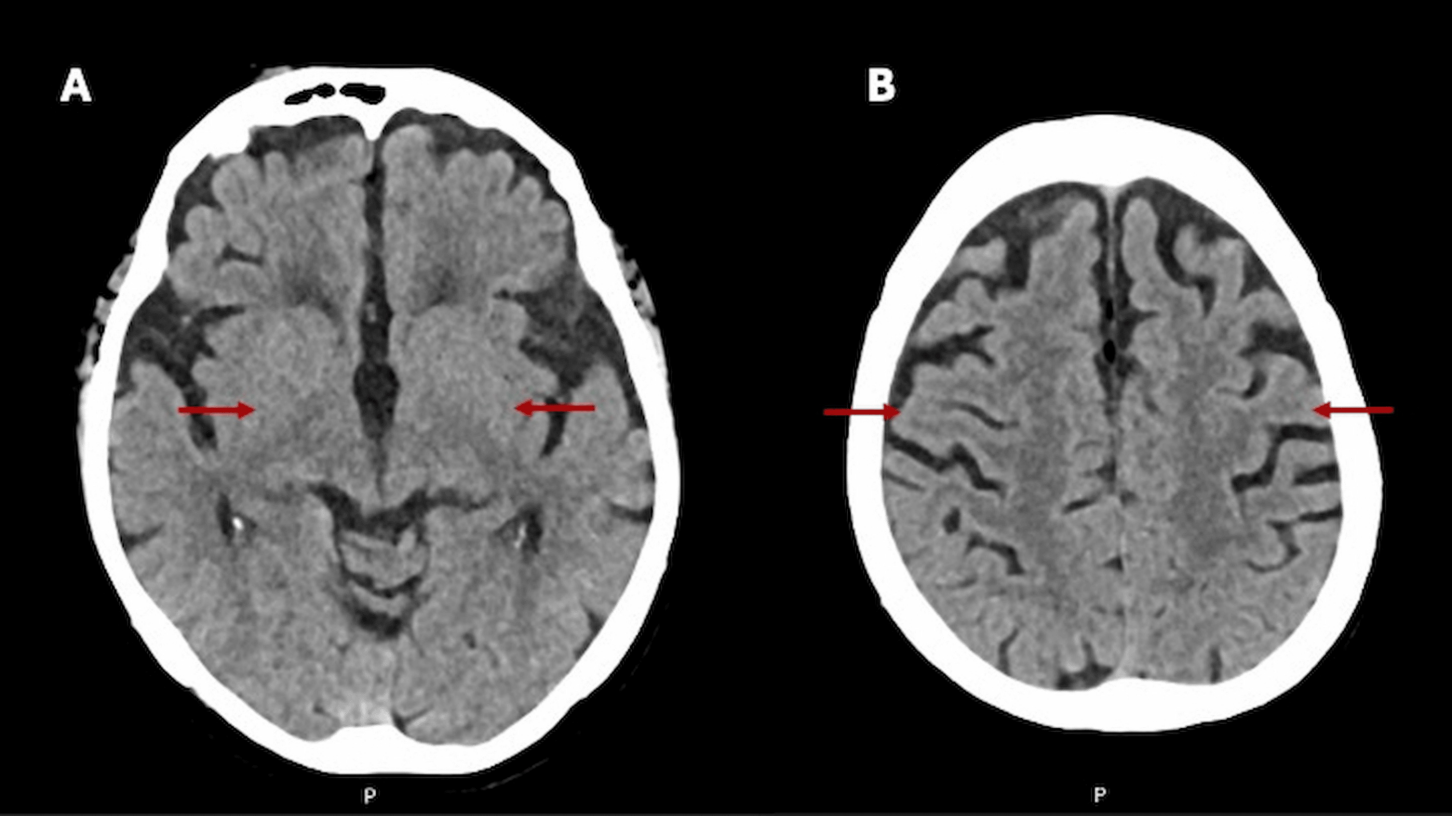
Axial non-contrast cerebral CT images revealing no acute pathology. (A) Cut at the level of the basal ganglia shows preserved symmetry, no hemorrhage, and no focal lesions. The arrow indicates the basal ganglia region, where no ischemic or space-occupying changes were detected. (B) Cut at the high convexity level demonstrates diffuse sulcal prominence, consistent with age-related cortical atrophy. No mass effect or midline shift is seen.
